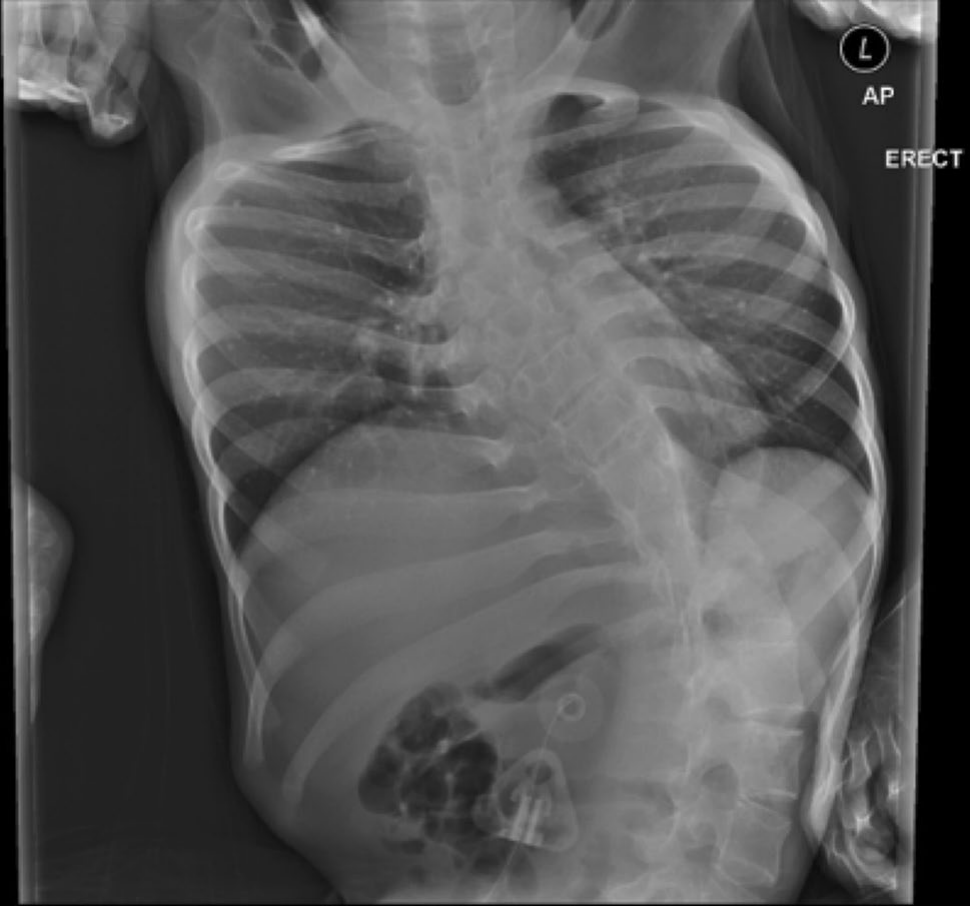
Lumbar spine x-ray image of patient with SMA Type-1 who has severe kyphoscoliosis
